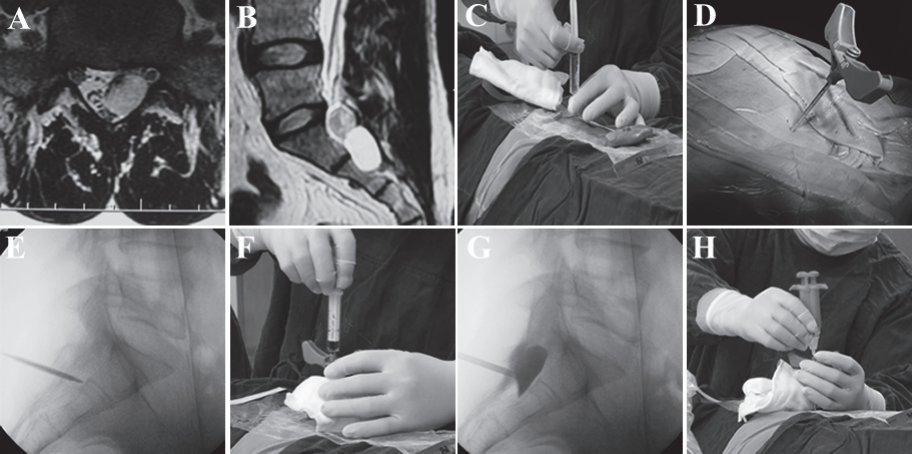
Procedure of fibrin gel therapy under C arm fluoroscopy machine guidance for symptomatic sacral perineural cysts. A and B. Transverse and sagittal T2WI MRI (prior to admission) show the location of cysts. C. Local anesthesia is induced with 2% lidocaine from skin to periosteum of sacral canal after needle insertion point is confirmed. D and E. Bone-puncture needle is used to puncture the sacral lamina and is inserted into the middle of the cyst guided by the C arm x-ray machine. F and G. CSF is aspirated and iohexol is injected to confirm the location of the bone-puncture needle in the cyst. H. Fibrin gel is injected into the cyst.
